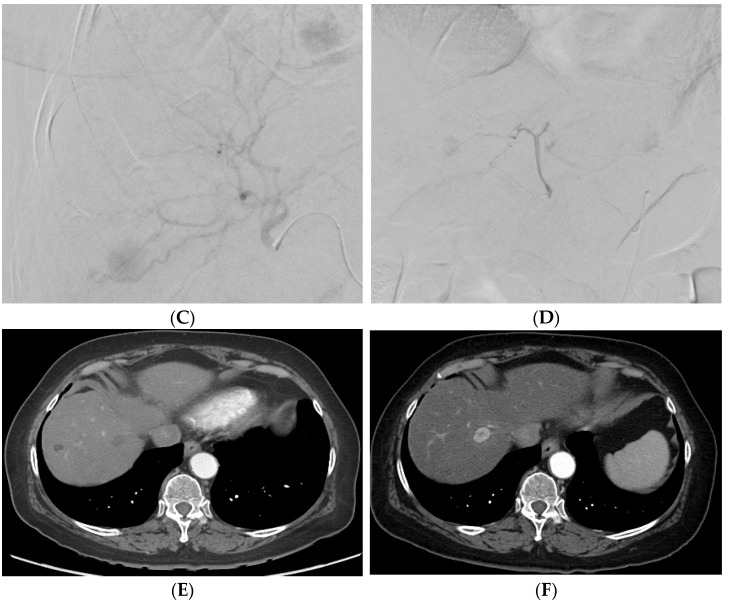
A patient with multifocal HCC who underwent DEB–TACE for its management. CT showing multifocal HCC involving the right (A) and left lobe (B). Common hepatic artery angiogram showing tumor blush (C), which completely disappeared after DEB–TACE (D). Follow-up CT showing good tumor response in the right (E) and left (F) lobe of the liver.
